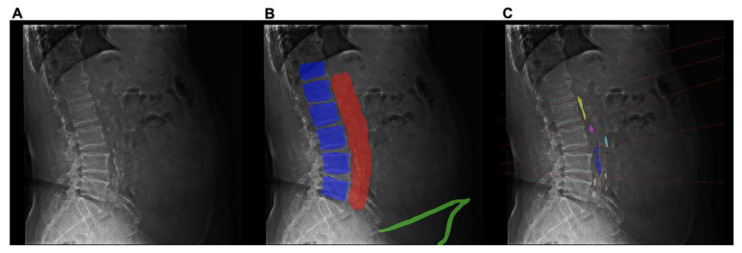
Example of the image annotation and quantification of the abdominal aortic calcification (AAC) Kauppila score in lateral lumbar radiographs. (A) The lateral lumbar radiograph of a 56-year-old man diagnosed with stage 5 chronic kidney disease. (B) The vertebrae T12–L5 (purple), aortic area (red), calcifications in the anterior (yellow) and posterior (blue) walls of the aorta, and peritoneal dialysis catheter (green) were annotated. (C) The AAC was scored following the Kauppila scoring system, with the boundary set as the midpoint of the intervertebral space above and below the vertebrae.
